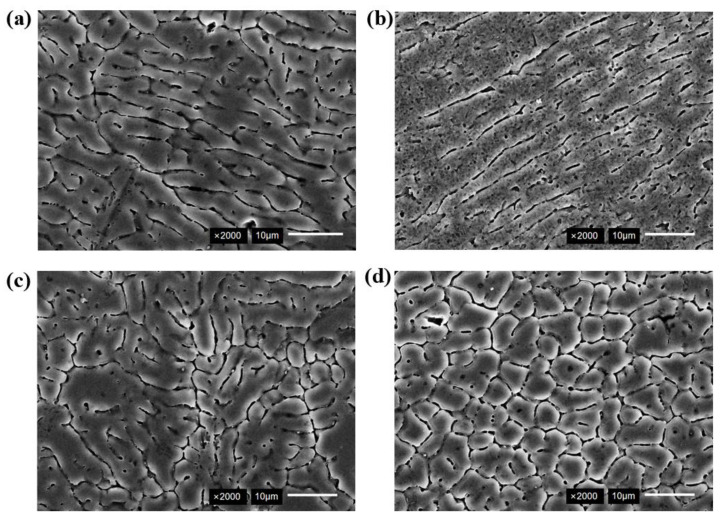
Fusion boundary zone: (a) no ultrasonic; (b) ultrasonic; (c) no ultrasonic; (d) ultrasonic.
